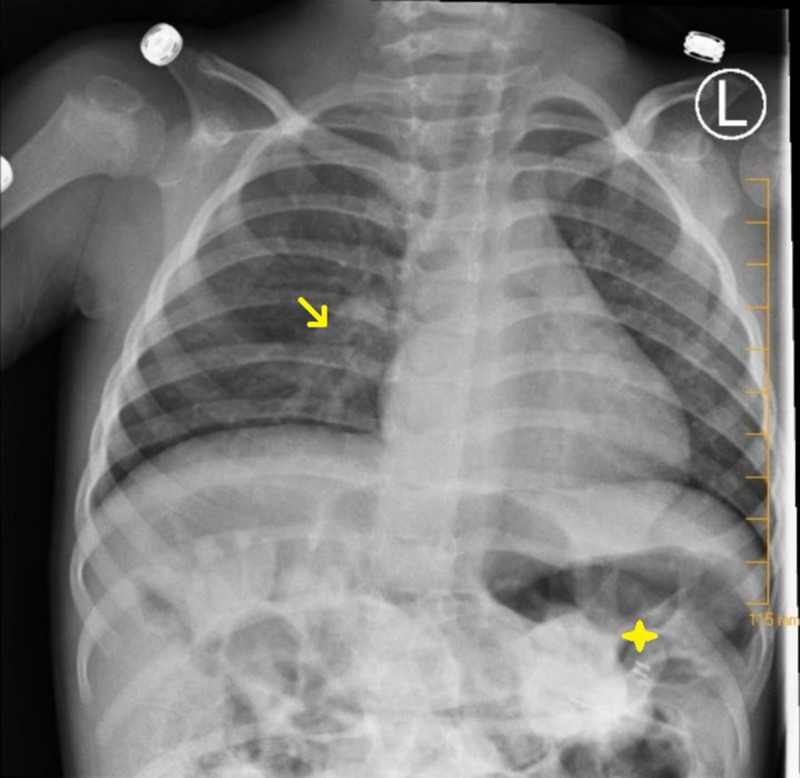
X-ray chest, PA view: mild increase in perihilar bronchial wall thickening and interstitial opacities suggestive of viral chest infection (arrow). No focal consolidation to suggest bacterial pneumonia. No pleural effusion or pneumothorax. Percutaneous gastrostomy tube visible (star). L, Left; PA, posteroanterior
